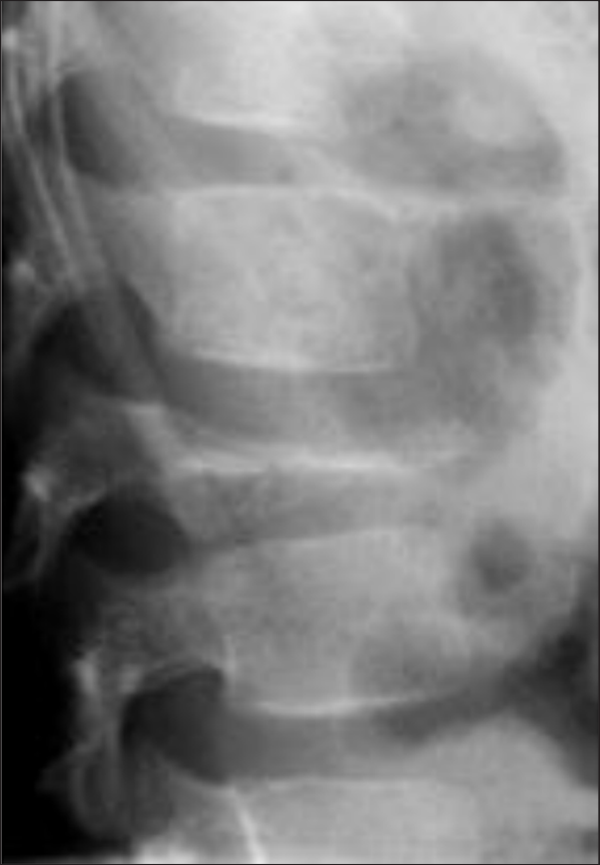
Eosinophilic granuloma (solitary form of Langerhans’ cell histiocytosis). Lateral radiograph shows collapse of the vertebral body L1 (vertebra plana).
